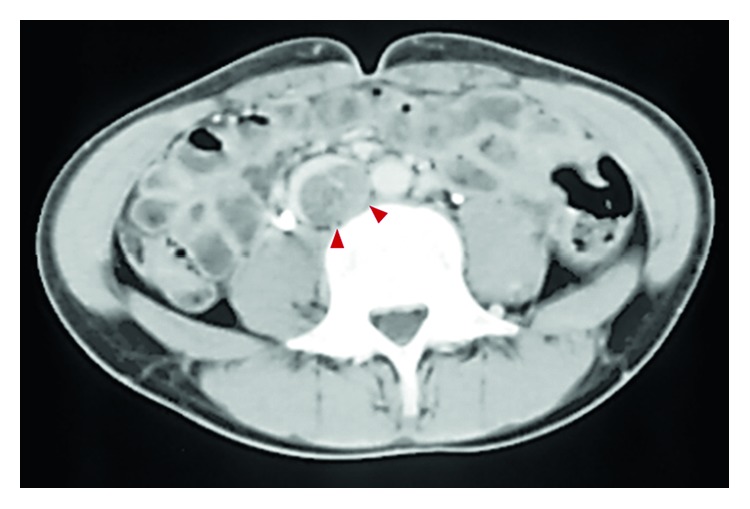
A contrast-enhanced CT suggesting the existence of the tumor thrombus of IVC.
